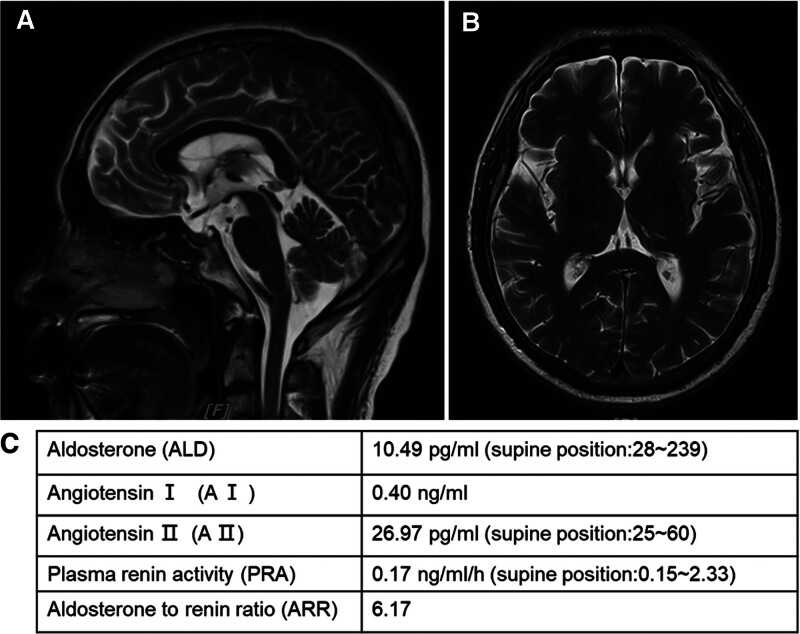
Patient’s brain MRI and hypertensive hormone testing after hypertensive emergencies. (A) Brain MRI showed Rathke’s cyst in the pituitary gland. (B) Brain MRI showed multiple lacunar infarctions in the thalamic region. (C) Renin–angiotensin system test showed no abnormalities.
